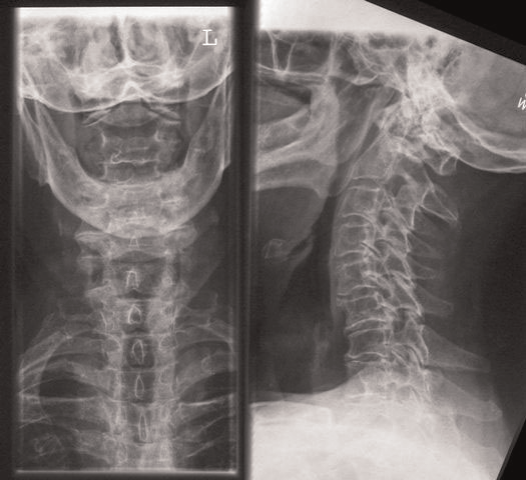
X-ray image of the cervical spine in two planes showing degenerative cervical spine disease with multi-level osteochondrosis. Odontoid process can not be seen.
